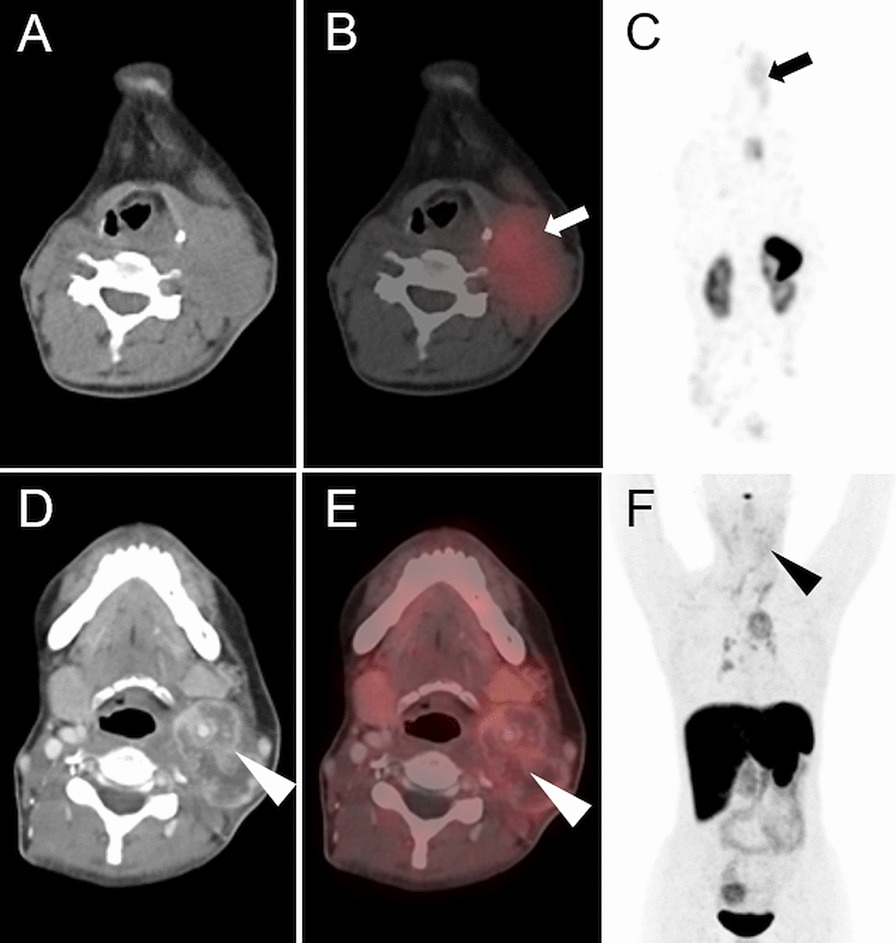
Effect of premedication on the [^177^Lu]Lu-PP-F11N uptake in patient 4. Axial CT, SPECT/CT and MIP of [^177^Lu]Lu-PP-F11N SPECT/CT (A–C) and [^68^Ga]Ga-DOTATOC PET/CT (D–F) of patient 4. Uptake of [^177^Lu]Lu-PP-F11N (arrows, with Entresto^®^ premedication) and [^68^Ga]Ga-DOTATOC (arrowheads) in the predominantly necrotic lymph node metastasis with only low intensity
